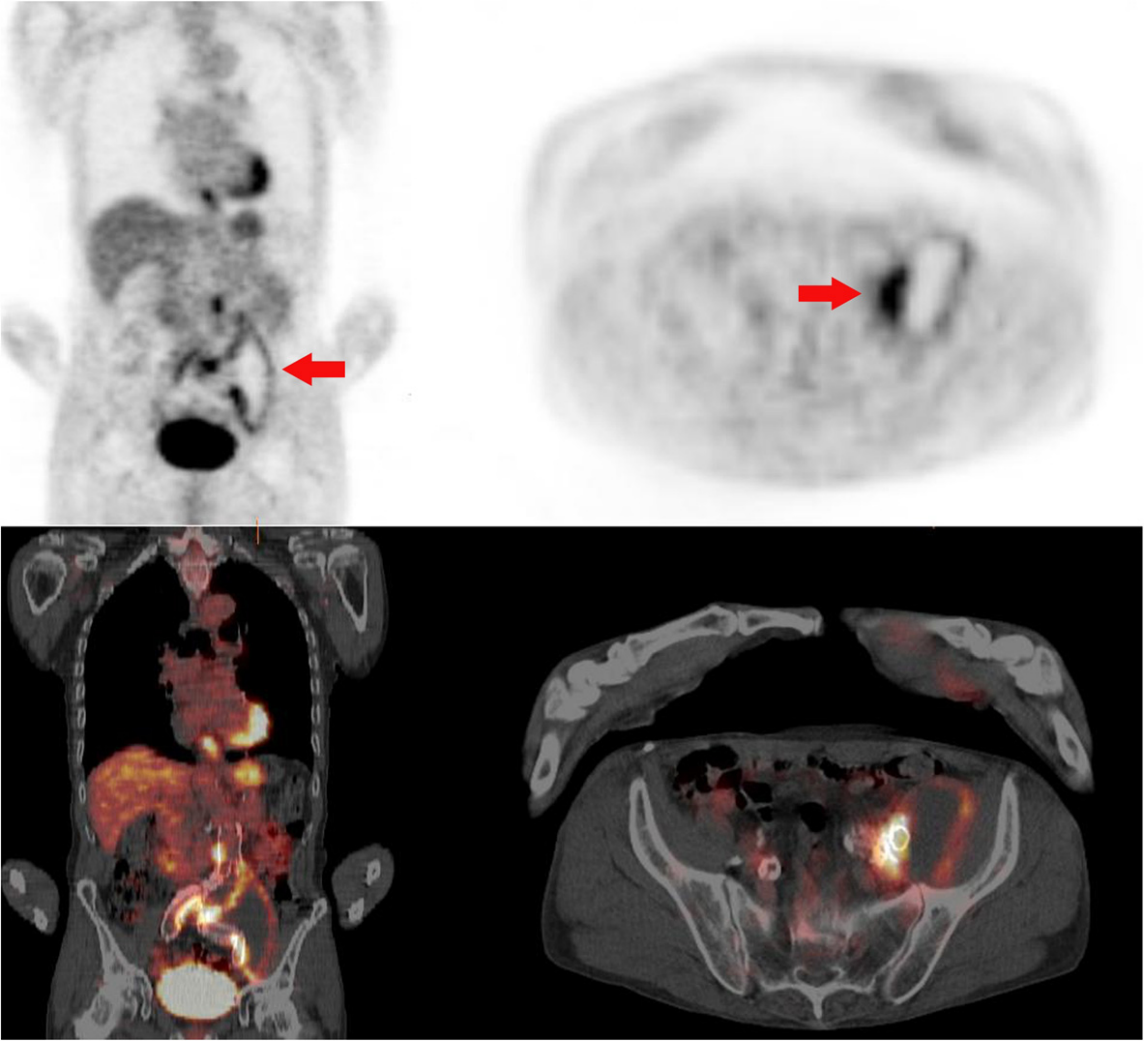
^18^F-FDG PET/CT image demonstrating a mycotic aneurysm. ^18^F-FDG PET/CT images (left column coronal sections, right column transverse sections, upper row PET images, lower row PET/CT fusion images) of a patient with proven chronic Q fever demonstrating a mycotic aneurysm and associated abscess adjacent to the left common iliac artery (arrows). Abbrevations: ^*18*^*F DG-PET/CT*^18^F- fluorodeoxyglucose positron emission tomography combined with CT.
